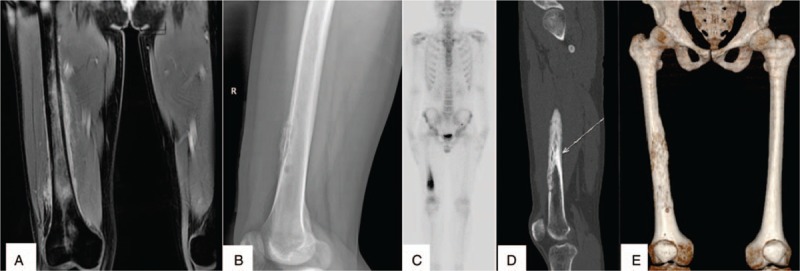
X-radiography, high-resolution computed tomography, and 3-dimensional reconstruction of the bone showing multiple lucent defects with a moth-eaten destruction of osteolysis in the right femur (A–C). Emission CT showed significantly increased uptake in the femur (D). Magnetic resonance imaging shows abnormal signal shadow in the femoral bone marrow cavity (E).
